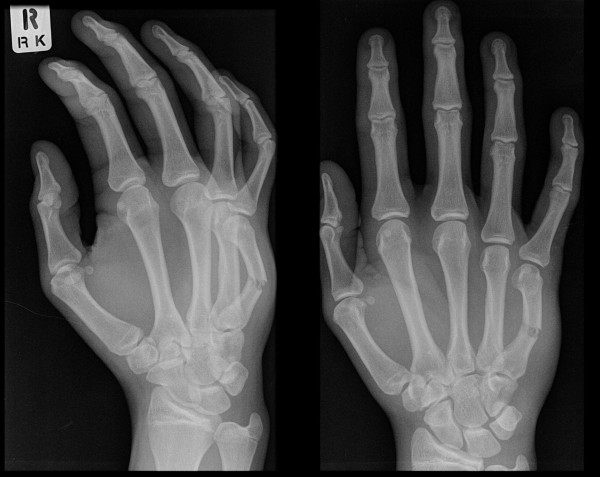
Pre-operative radiograph of an angulated little finger metacarpal shaft fracture in a skeletally mature adult.
